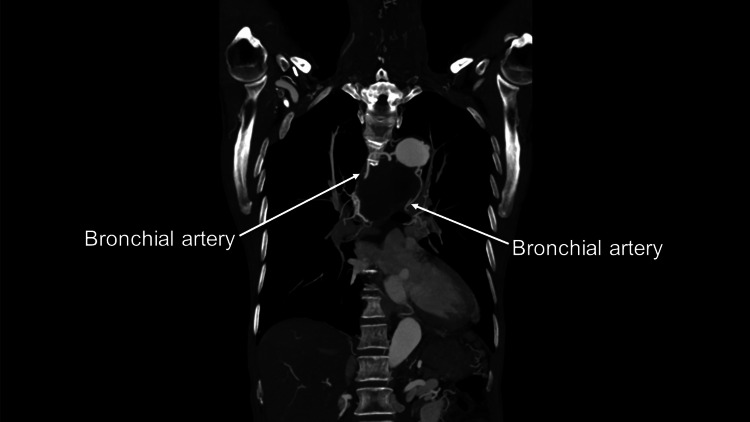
Preoperative contrast-enhanced computed tomography (CT) Better visualized bronchial arteries in the coronal view
